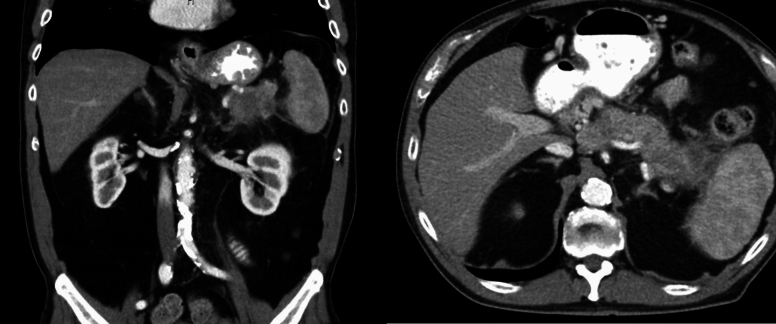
CT done 2 months later showing interval progression in phlegmonous changes around pancreatic tail and body with increase in surrounding inflammatory fat stranding
